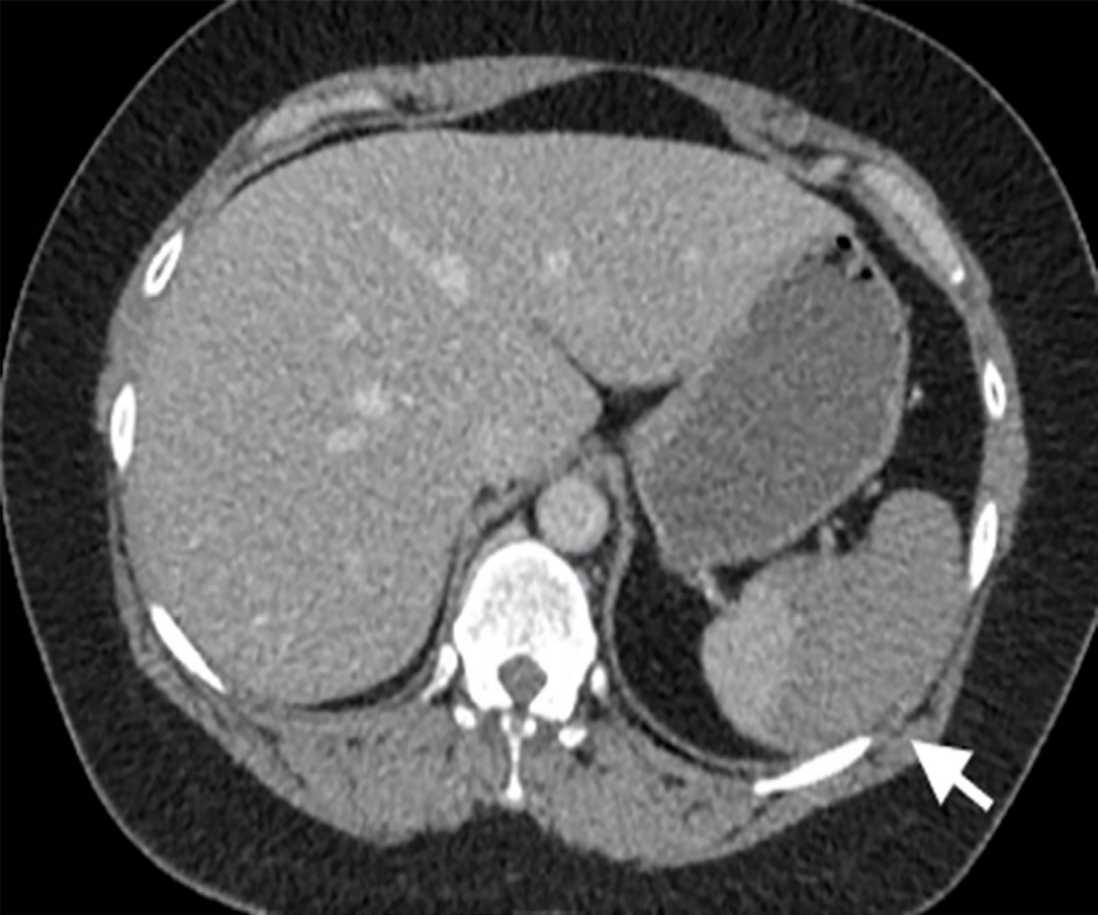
Computed tomography of the abdomen showing decreased contrast uptake in the spleen consistent with large area of infarct (arrow).
